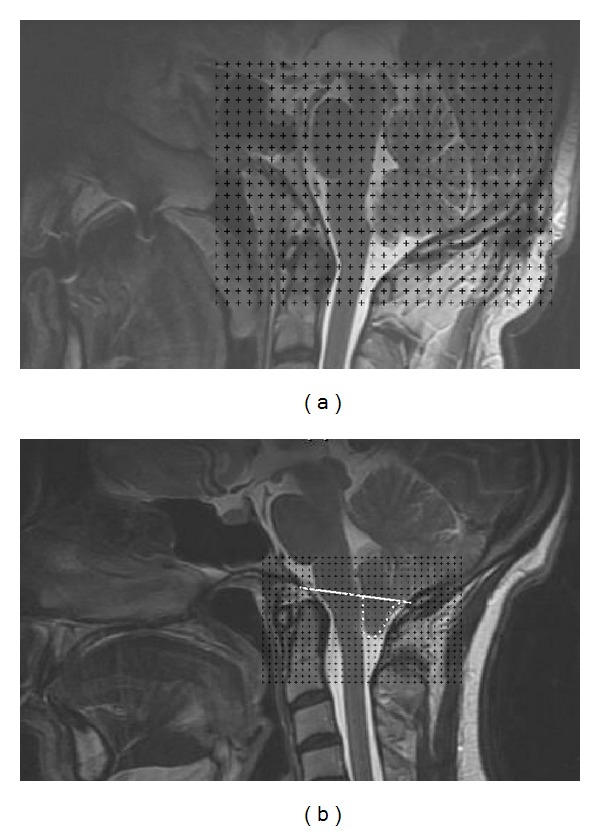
Representative midsagittal MRI for the cranial fossa and cerebellum (a) and herniated tonsils (b) with a grid overlaid for the calculation of volumes using the Cavalieri method.
